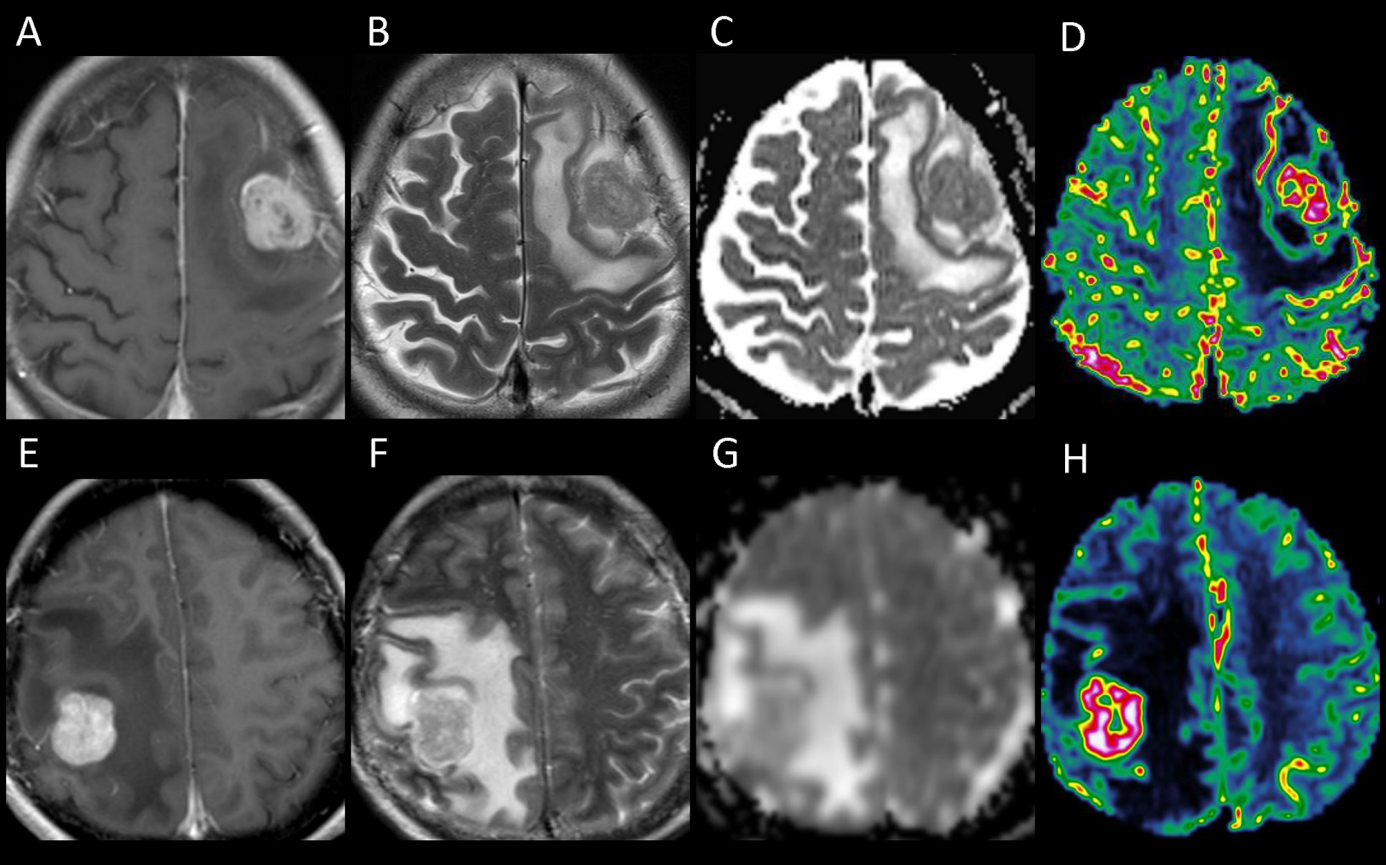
The upper panel shows the contrast-enhanced T1-weighted (A), T2-weighted (B), apparent diffusion coefficient (ADC) (C), and cerebral blood volume (CBV) (D) images of a left frontal metastasis with peritumoral brain edema (PTBE) of 85.98 cm^3^, ADC value of 817.9 × 10^-6^ mm^2^/s, and relative CBV of 4.37. The lower panel (E–H) shows the corresponding images from a right parietal metastasis with PTBE of 133.63 cm^3^, ADC value of 1,185 × 10^-6^ mm^2^/s, and relative CBV of 17.37. The ADC and CBV of the right parietal metastasis with a larger PTBE volume are higher than those of the left frontal metastasis which has a smaller PTBE volume.
